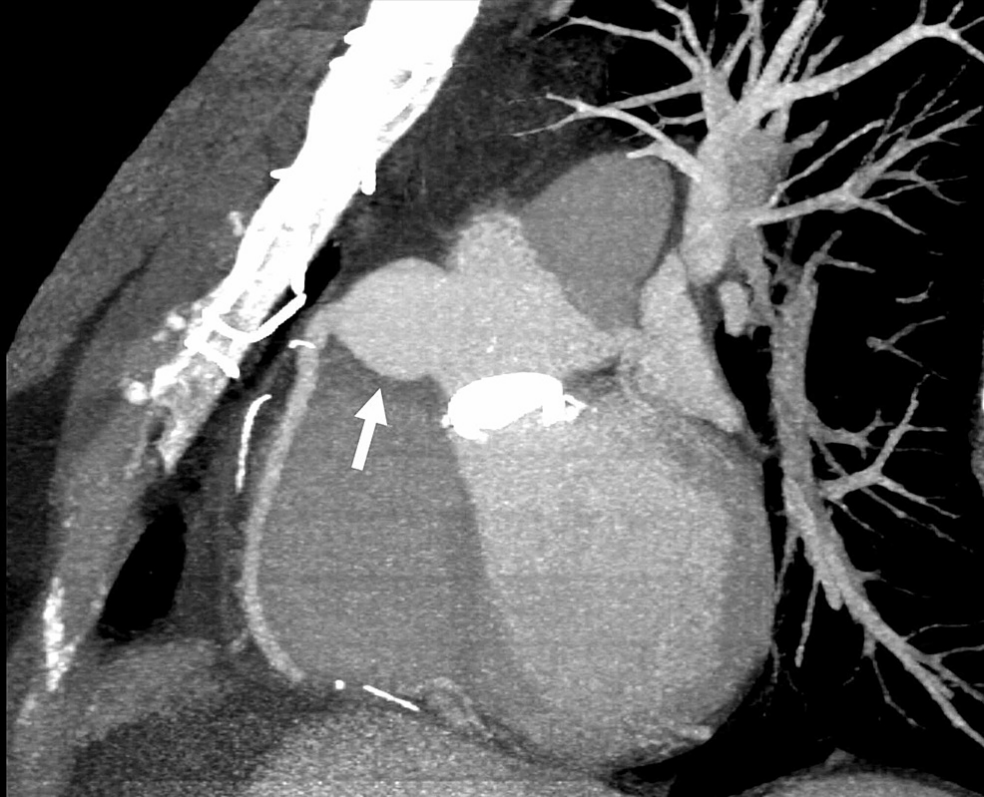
Maximum Intensity Projection Sagittal CTA Image Origin of the right coronary artery with aneurysmal dilatation of the first 3.5cm of the right coronary artery, which measures as much as 3.1cm in diameter (white arrow).
